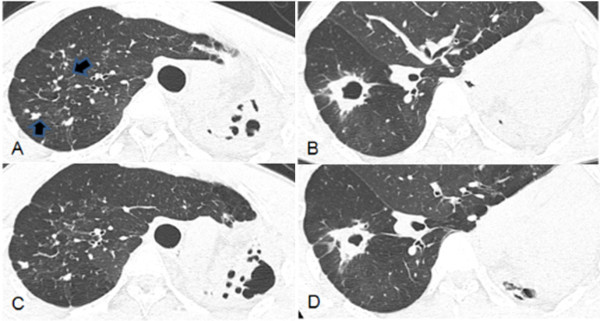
A, B, C, D Extensively drug-resistant tuberculosis in a 33-year-old man. A and B. Transaxial thin-section CT scan shows multiple centrilobular nodules (arrows) in the right upper lobe (A) and a cavity in the right lower lobe (B). Also note destroyed left lung with mediastinal shifting. C and D. After 8 months of linezolid treatment, CT scan shows partial improvement of pulmonary nodules in the right upper lobe (C) and the decrease of cavity size in the right lower lobe (D).
